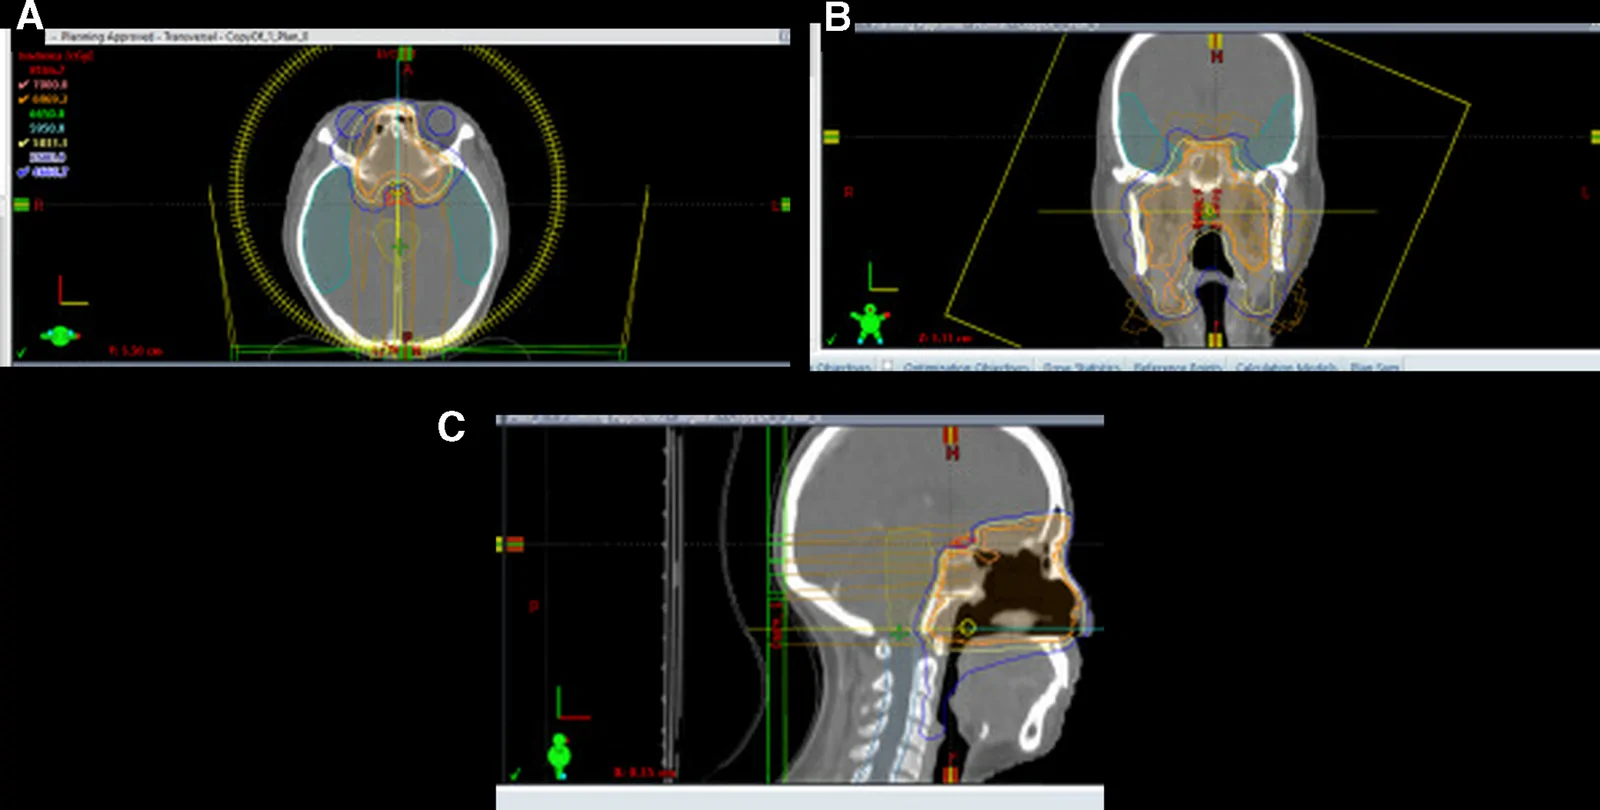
Postoperative VMAT/IMRT plan. (A–C) Representative axial, coronal, and sagittal planning CT images demonstrate target contours and dose distribution for the postoperative sinonasal and skull-base-related treatment volume. The final prescription was 70 Gy in 35 fractions. CT = computed tomography, IMRT = intensity-modulated radiotherapy, VMAT = volumetric modulated arc therapy.
